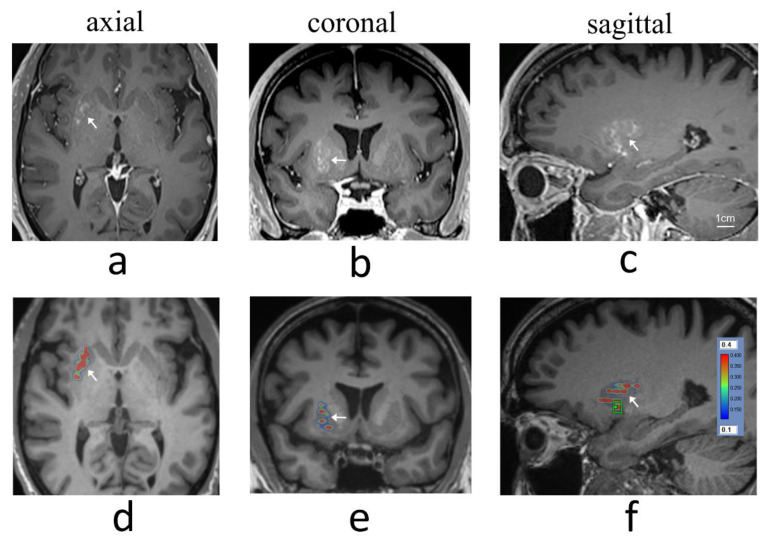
Gd-enhanced T1-weighted MRI showing BBB opening within the right putamen (arrows) immediately post-treatment in (a) axial, (b) coronal, and (c) sagittal views for treatment session 1 in patient 2 (cavitation dose target = 0.40). Images (d–f) show corresponding cavitation dose maps overlaid on pre-treatment T1-weighted planning MR images (green box in image (f) indicates the final sonication grid).
